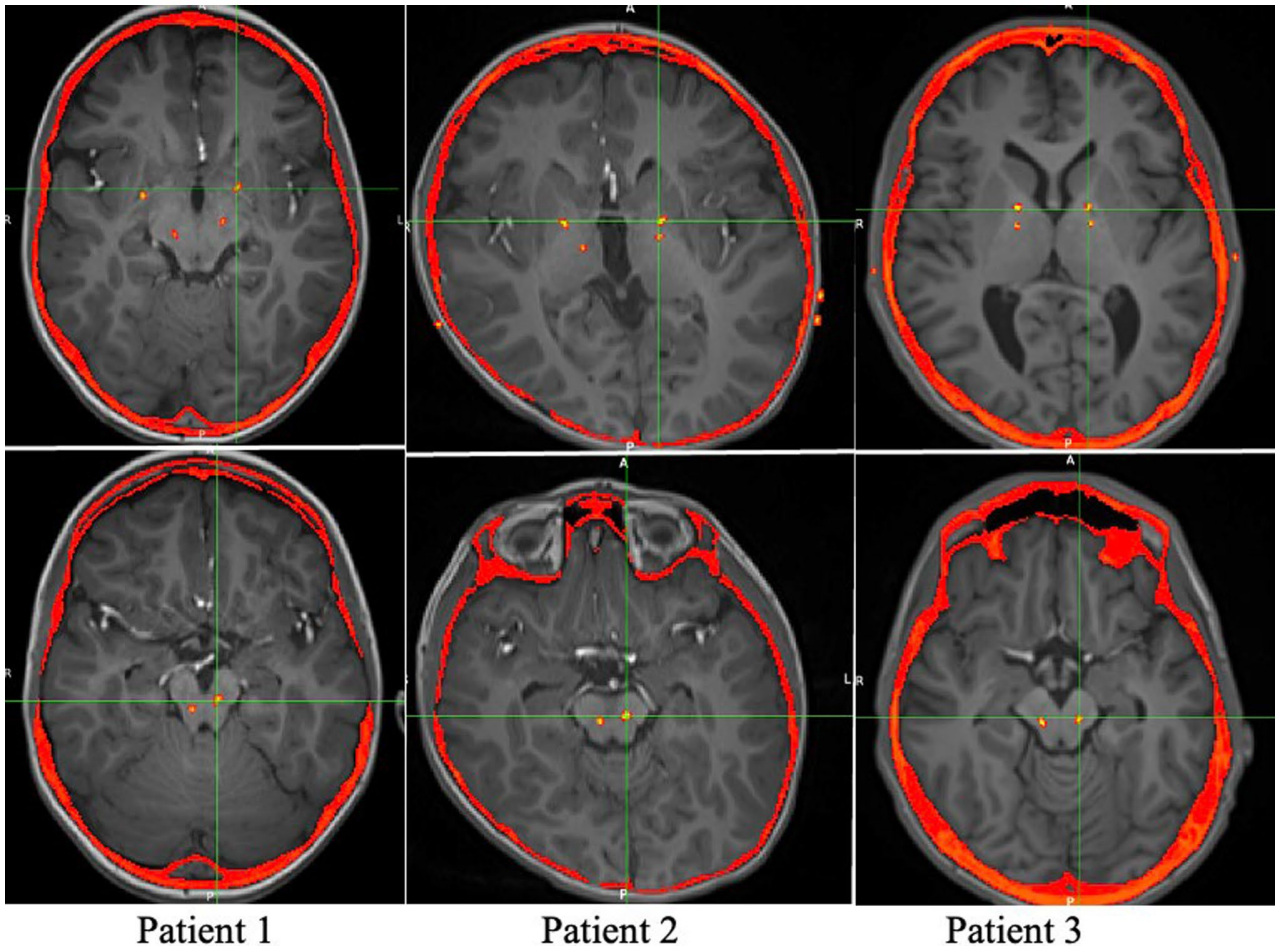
Axial views of the postoperative CT overlaid on the preoperative MRI, showing the locations of the Medtronic leads.
